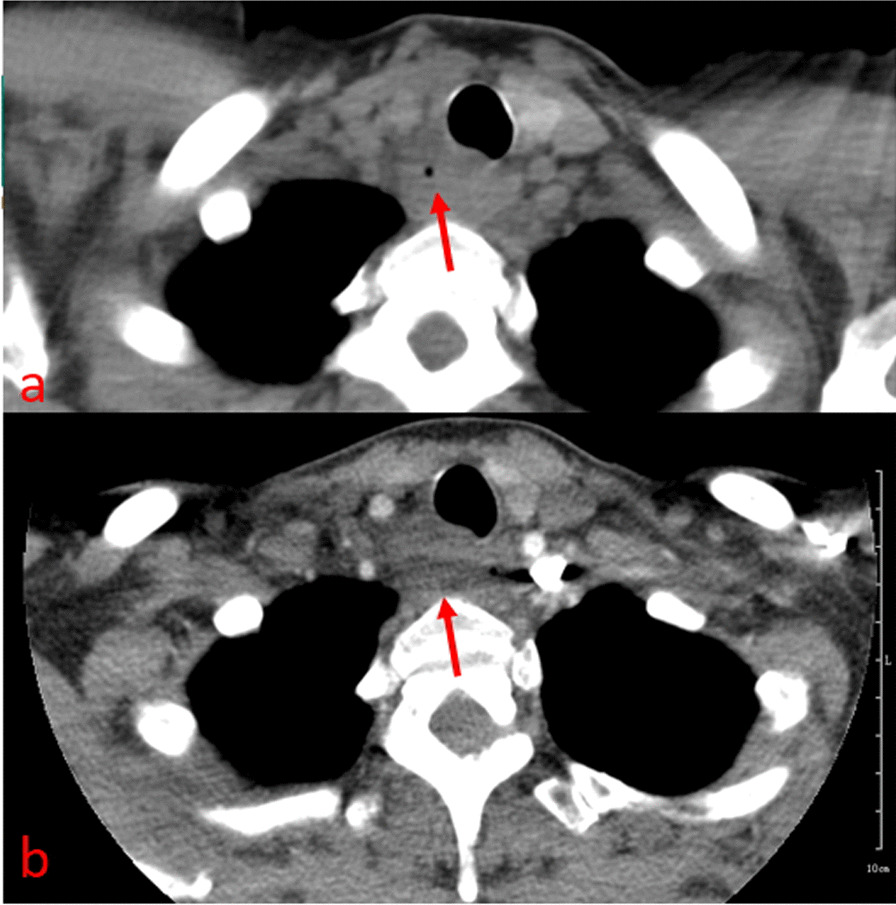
Axial CT image (a) showing a lower-density mass just behind the upper trachea (arrow). The small bubble inside the lesion suggests it may be an abscess. No enhancement was found after contrast injection (b). CT could not identify the origin of the lesion
